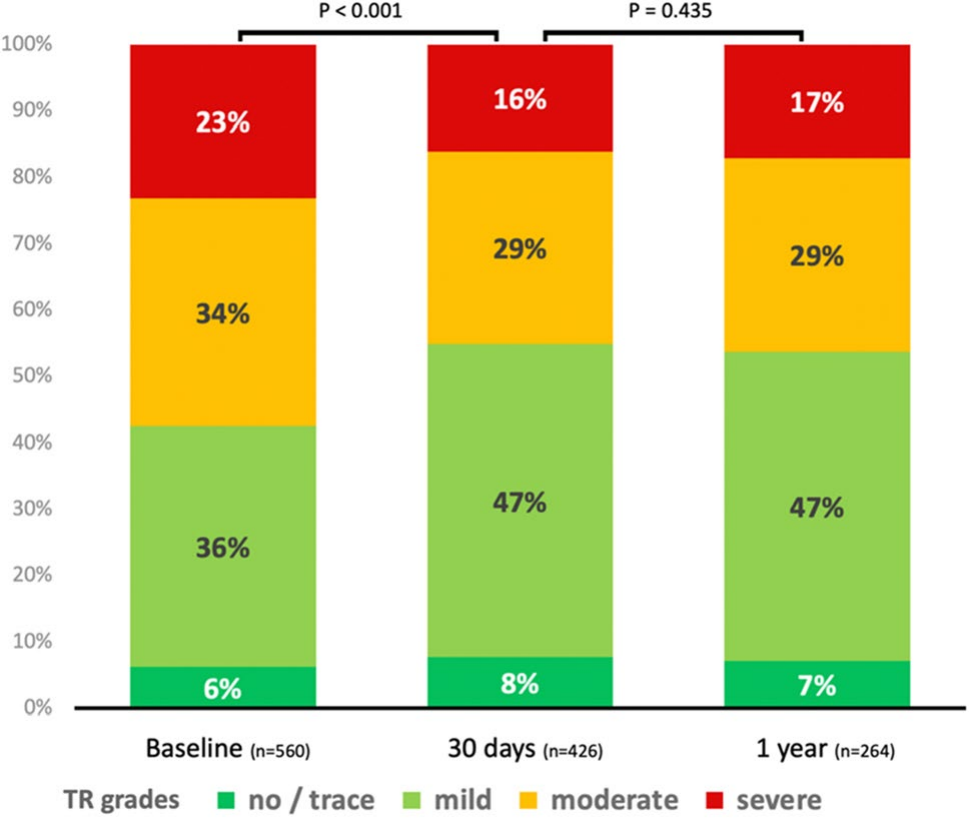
Changes in concomitant Tricuspid Valve regurgitation severity grades from baseline to 1-year follow-up. Post-interventional reduction of Tricuspid Regurgitation (TR): echocardiographic assessments at baseline, 30 days and 1 year
